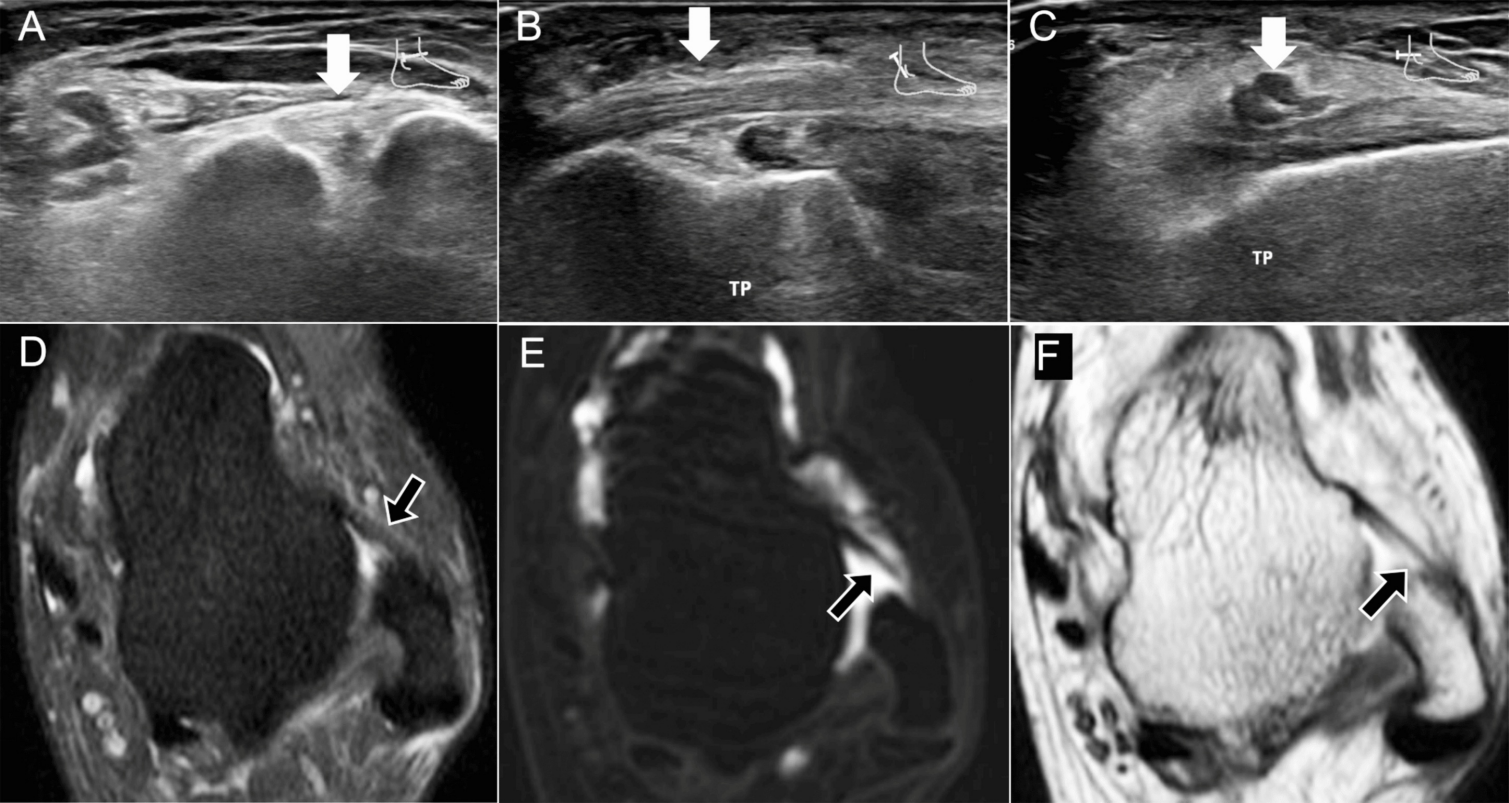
Ultrasound and MRI of ATFL tear with intact peroneal tendons. A 32-year-old female with chronic instability: (A) transverse ultrasound shows a full-thickness (Grade III) ATFL tear (arrow); (B, C) longitudinal and transverse ultrasound at the retromalleolar level show intact peroneal tendons (arrows); (D) pre-contrast DPFS MRI shows a thickened, hyperintense ATFL (arrow); (E) post-contrast T1 SPGR MR arthrography (spoiled gradient-echo) shows loss of fibular insertion continuity (arrow); (F) post-contrast T1 spin-echo MRI confirms an ATFL tear with a few residual fibers (arrow). AFTL: anterior talofibular ligament; DPFS: dual proton fat-saturated sequence; SPGR: spoiled gradient-echo Images belong to the authors; patient consent was obtained.
